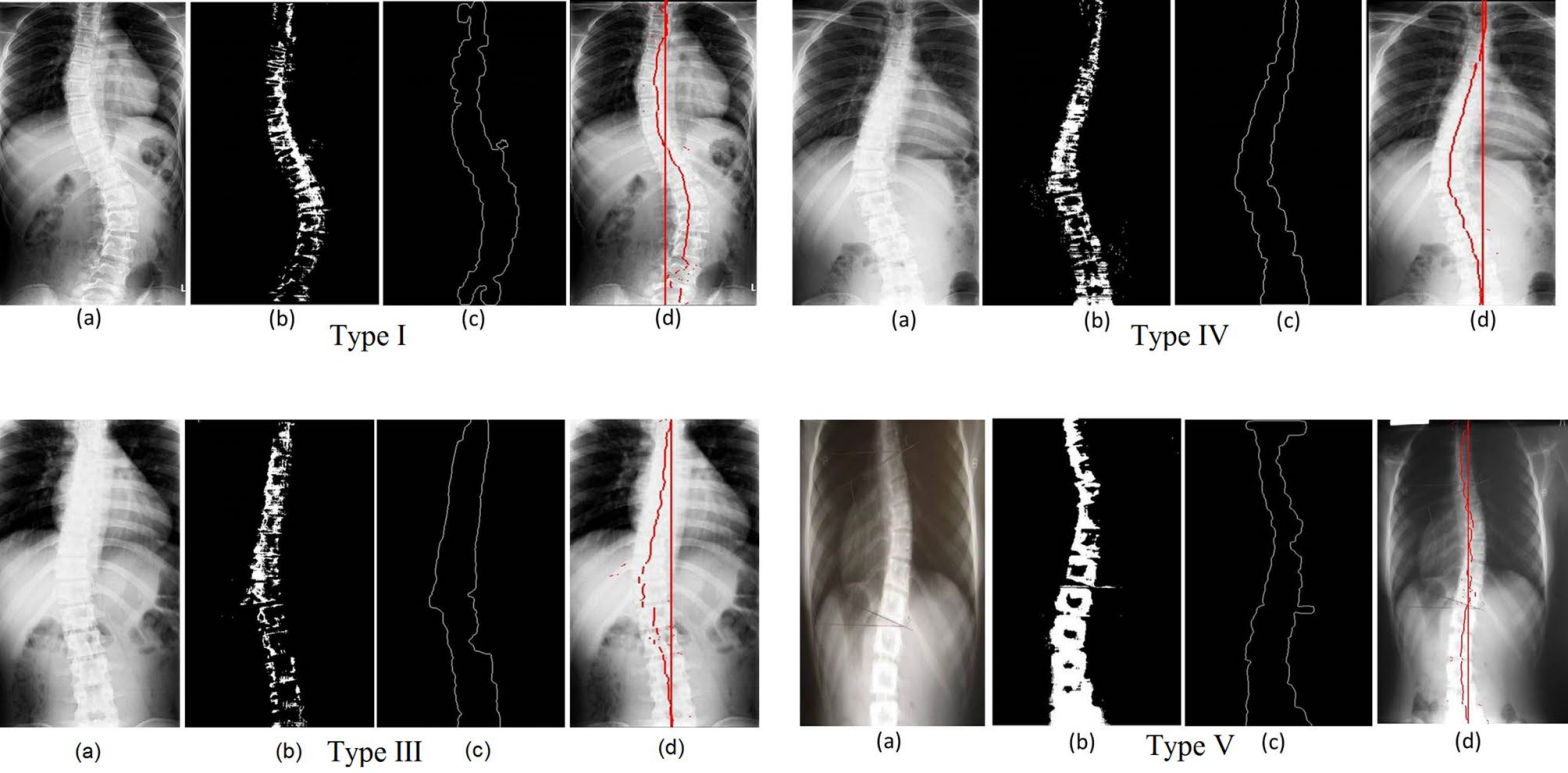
Images of Type-I, Type-III, Type-IV and Type-V for (a) input radiograph, (b) segmented vertebral column, (c) extracted boundary and (d) automated definition of MA and CSL.
